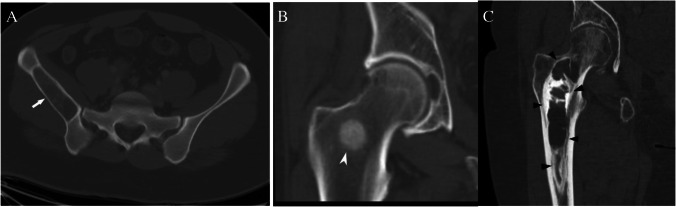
CT classification of bone lesions by density. CT images show A lucent (arrow), B sclerotic (pointed arrow), and C mixed density (arrowheads) lesions in 3 patients with giant cell tumor, prostate cancer metastasis, and fibrous dysplasia, respectively
